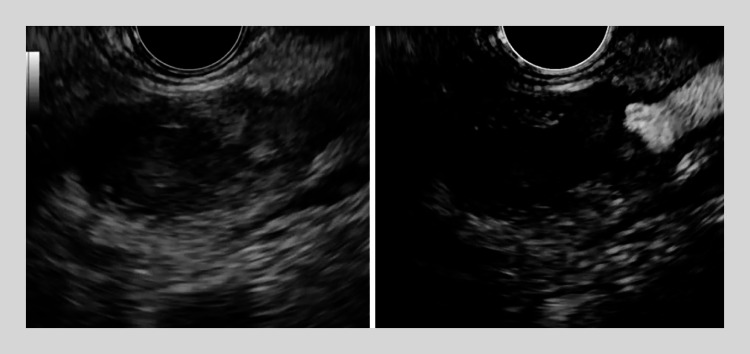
Contrast-enhanced endoscopic ultrasound images showing no vascularity within the tumor-like lesion and a splenic artery aneurysm, so the tumor-like lesion was considered to be coagulum.
